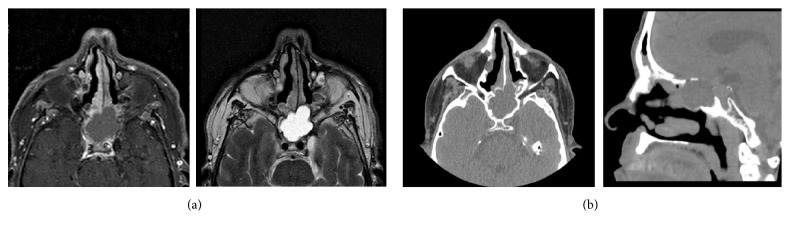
Neuroimaging of the sphenoid sinus mucocele. (a) Paranasal sinus MRI revealing molding of the medial wall of left cavernous sinus by the sphenoid mass. (b) Paranasal sinus CT scan showing sclerosis and interruption of the roof and posterior wall of left sphenoid hemisinus.
